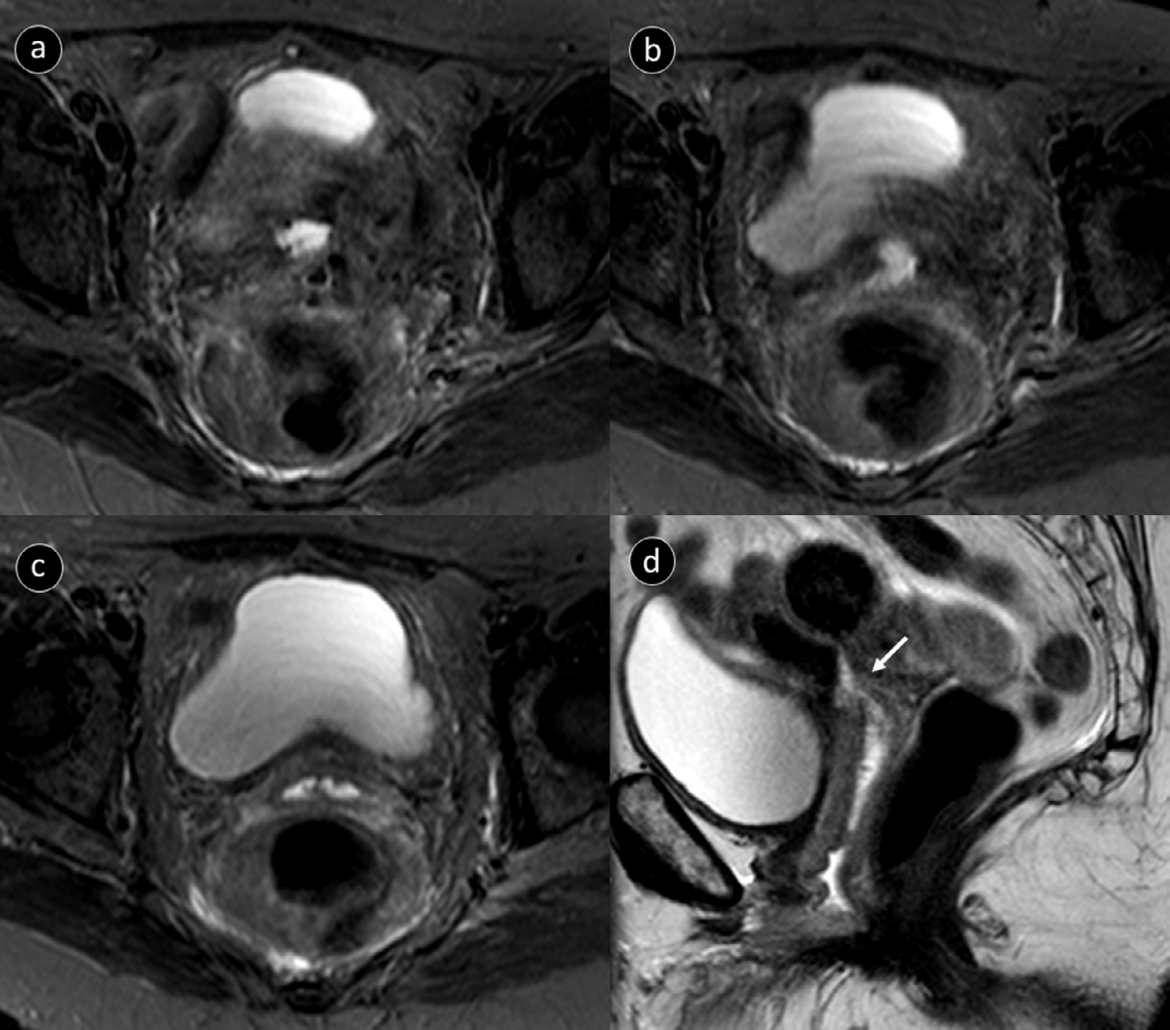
MRI study performed after admission (a, b, c) sequential axial STIR images demonstrating fluid within the small bowel, a fistula containing fluid and fluid within the vagina, respectively. (d) sagittal *T_2_* weighted image demonstrating clearly the fistulous connection (arrow) between the superior vagina and small bowel. STIR, short-tau inversion recovery.
